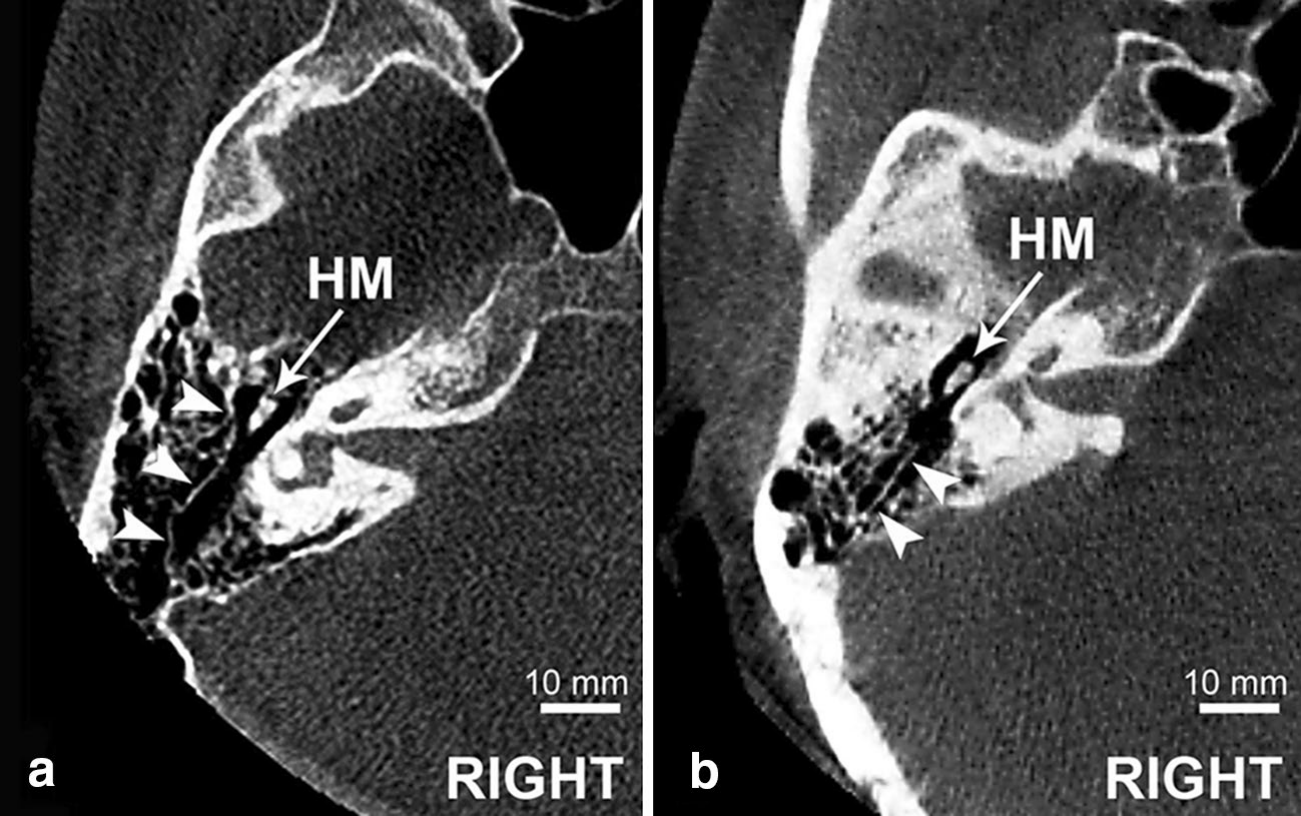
Axial CBCT scans at the level of HM presenting complete (a) and incomplete KS (b). KS marked with arrowheads
